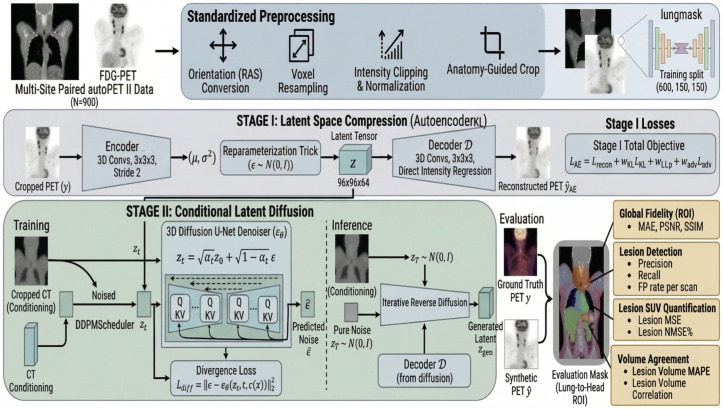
Overview of the proposed conditional 3D latent diffusion (3D-LDM) for CT-to-PET translation in the head–neck and thoracic region.
